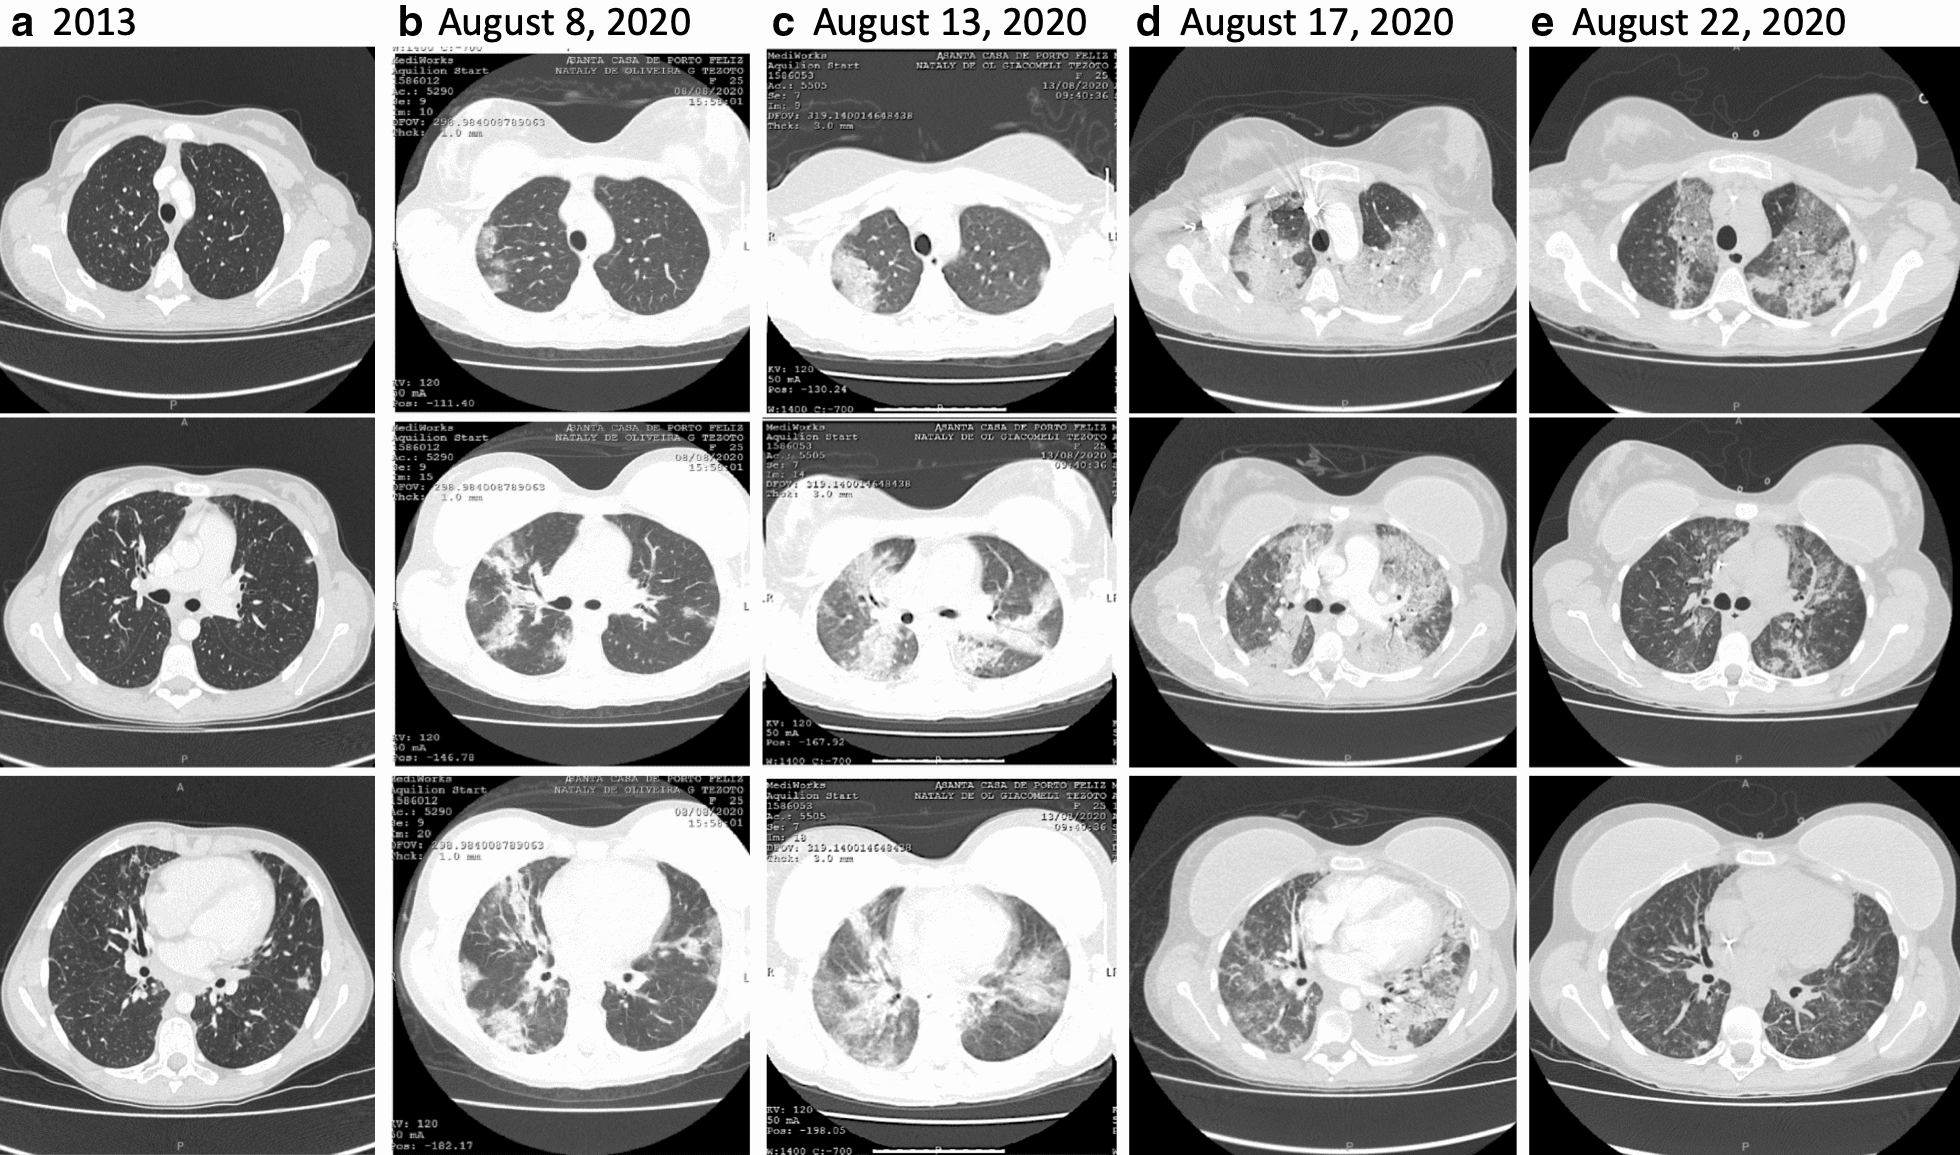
Lung computed tomography scans obtained at the time of common variable immunodeficiency diagnosis, in 2013 (a) and during COVID-19, (b–e). The dates when scans were performed are depicted in the Figure. As for the interpretation of scans, the patient was submitted to a breast implant in 2017
